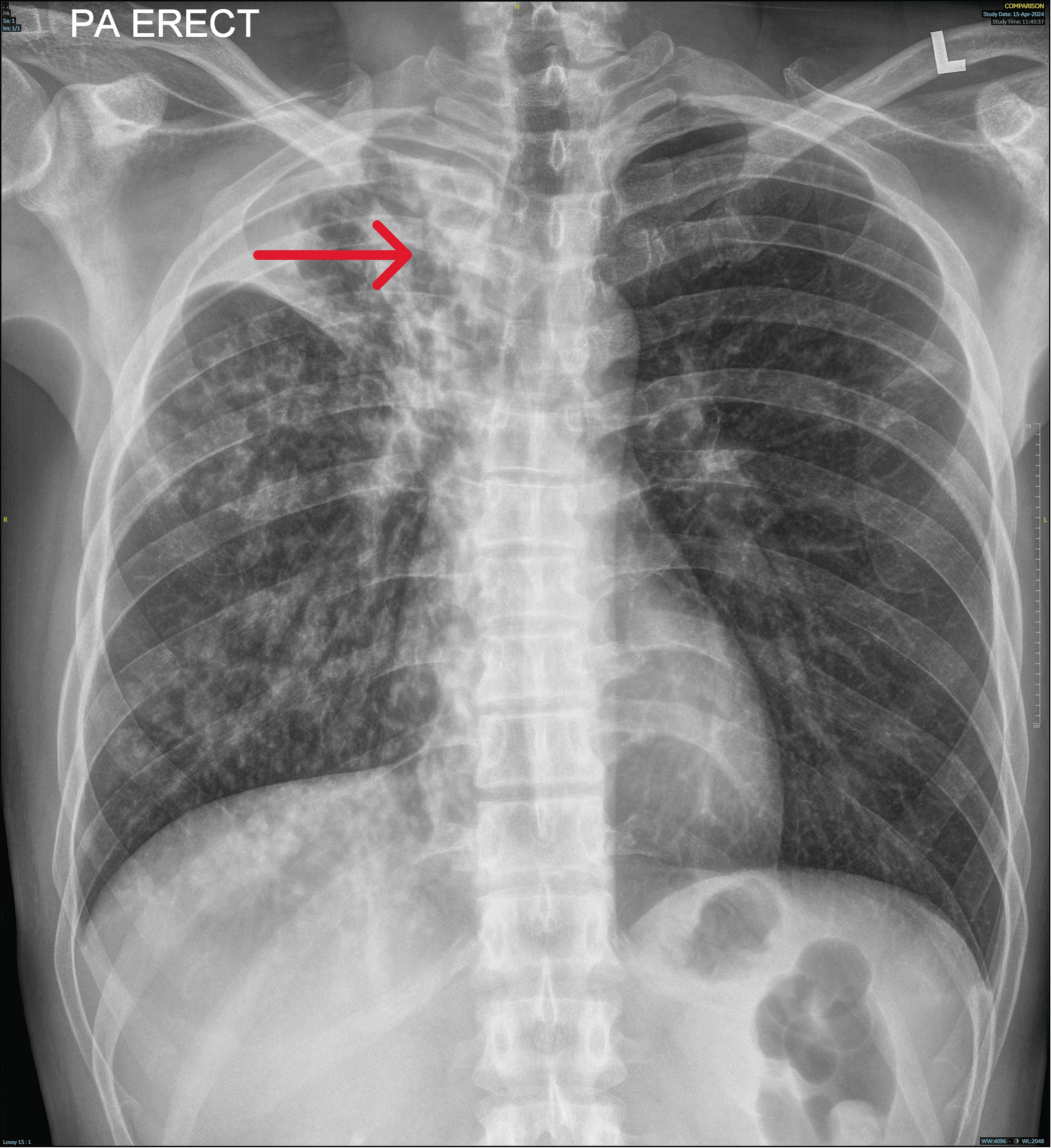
Chest radiograph showing right upper lobe and middle lobe lung opacities (red arrow)
